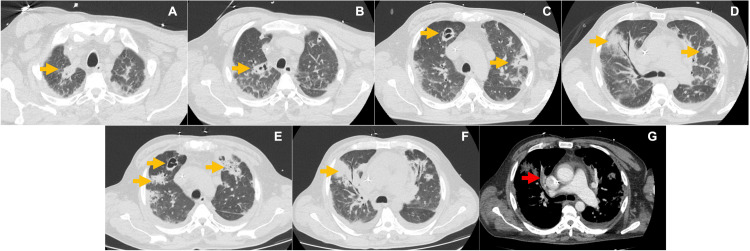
Temporal evolution of lung CT scans showing progression of pulmonary consolidations. (A-D) Day nine after admission. (E-G) Day 18 after admission. Yellow arrows indicate multiple septic consolidations, with some nodular cavitations and the red arrow indicates a filling defect of the middle lobar artery.
